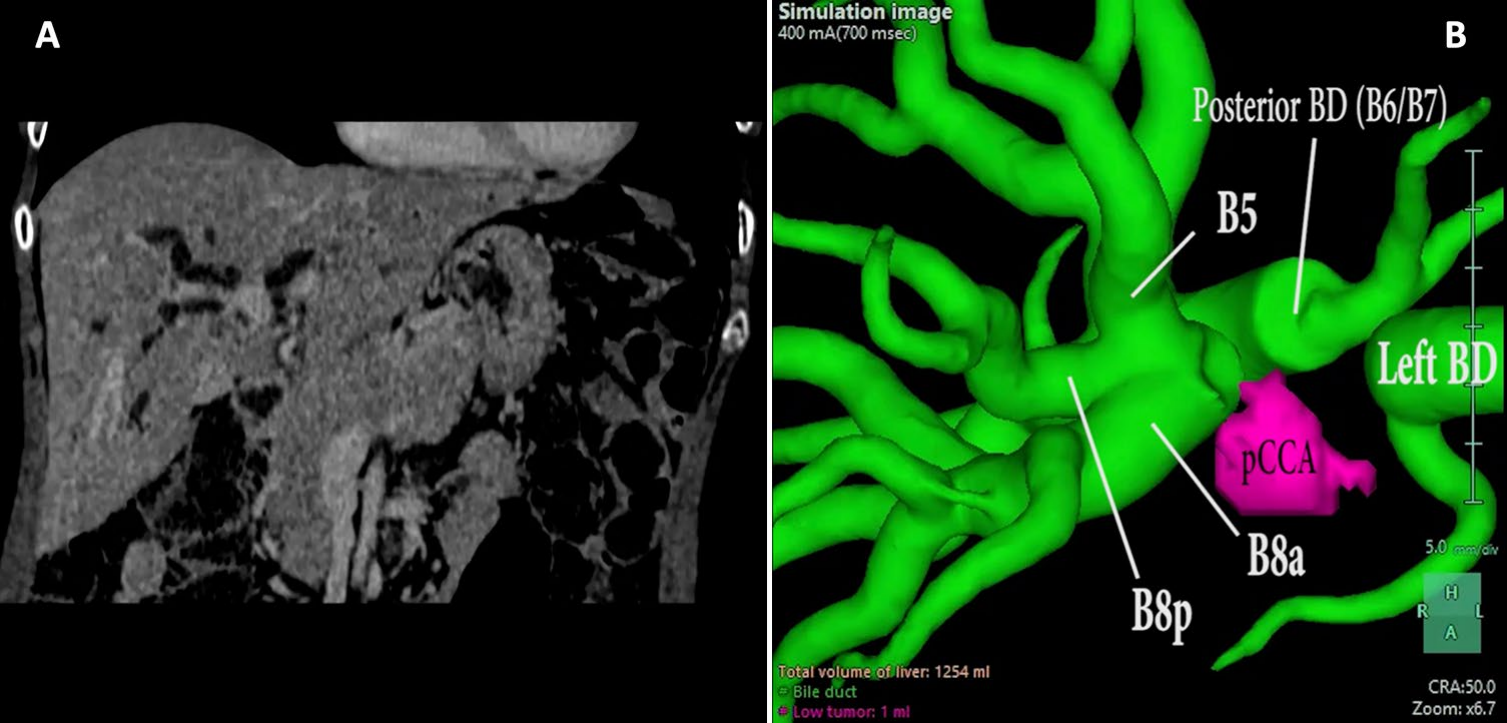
Preoperative CT scan of patient 4 (a); 3D reconstruction of the biliary tree of patient 4 (b)
